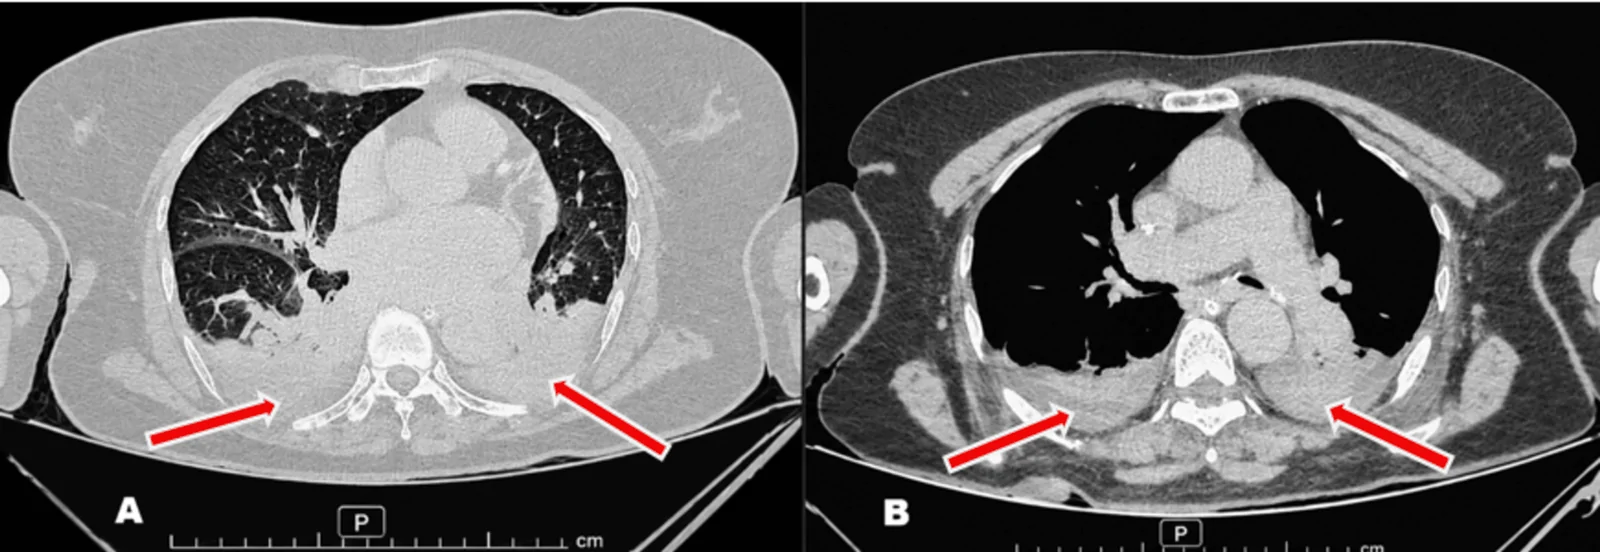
Computed tomography (CT) of the thorax on day 2 post-admission demonstrating pulmonary and mediastinal findings. (A) Lung window image showing mild bilateral pleural effusions with dependent bibasal atelectatic-consolidative changes (arrows). (B) Axial CT image in the mediastinal window demonstrating no evidence of tracheal compression, airway narrowing, or other mediastinal pathology (arrows indicate the pleural effusions).
